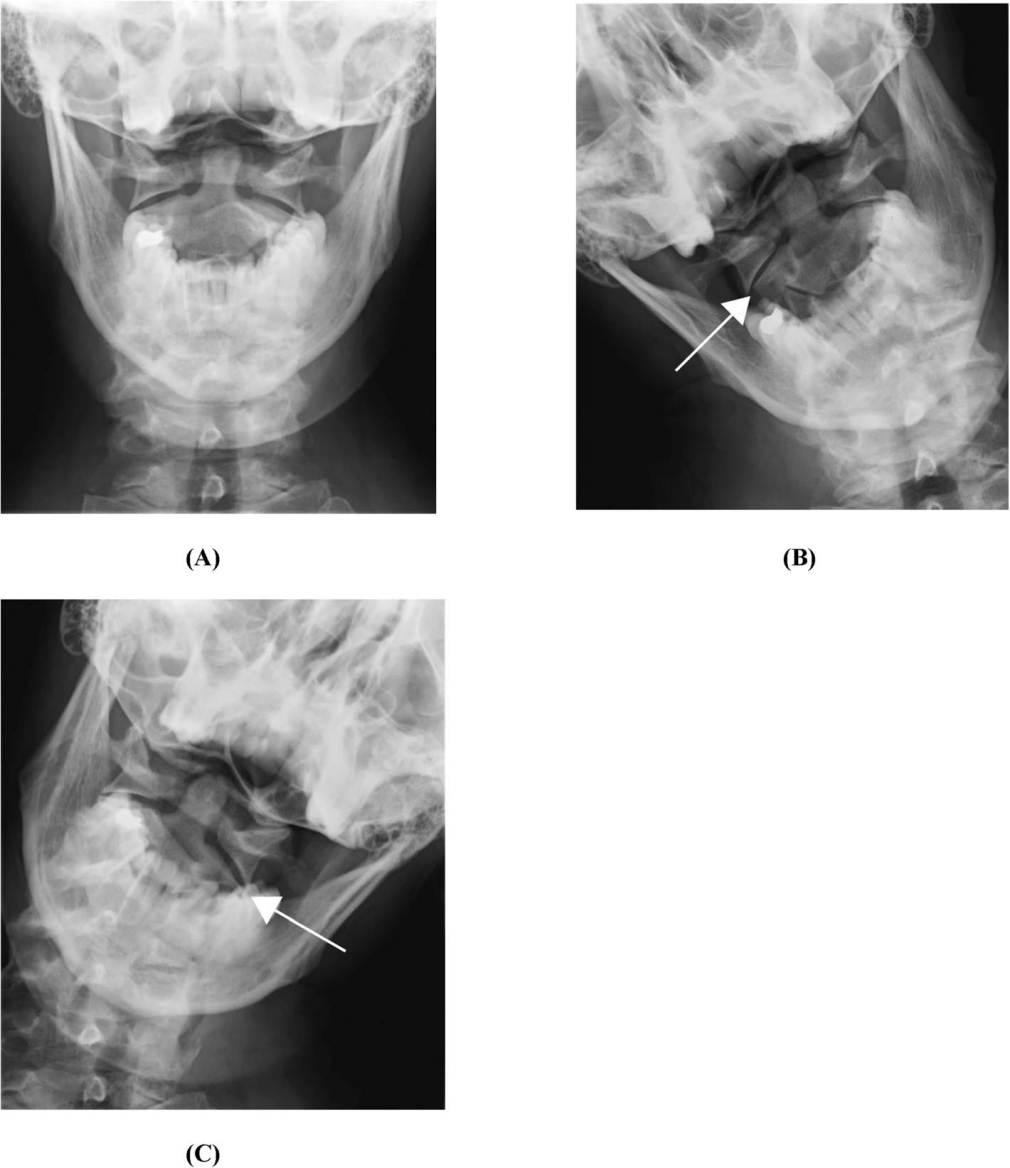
Open mouth Anteroposterior Lateral bending cervical spine radiographs in neutral (a); right side bending (b); left side bending (c) [20]
